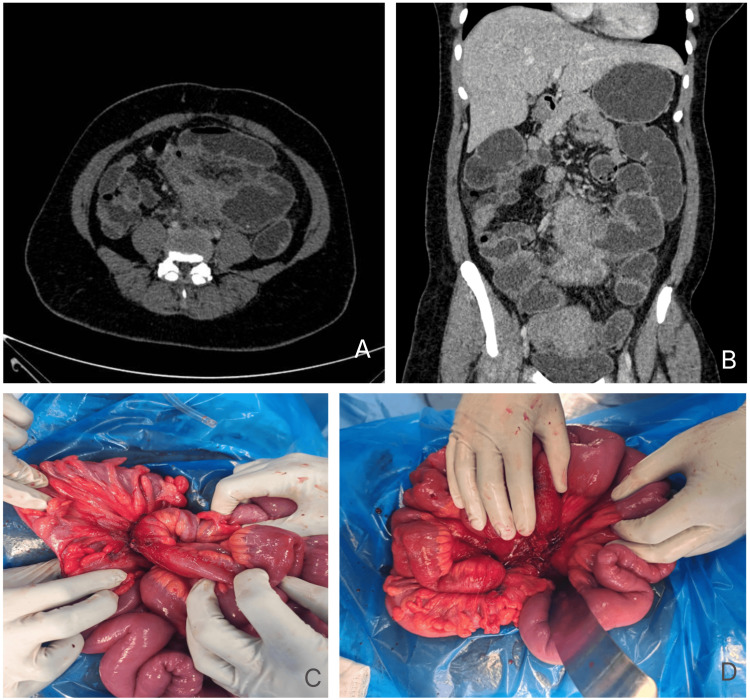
(A and B) CECT scan of the abdomen showed diffuse mural thickening involving a mid-ileal segment of approximately 6 cm, accompanied by luminal narrowing, surrounding inflammatory fat stranding, and an adjacent heterogeneously enhancing spiculated mass measuring 3.8 x 4.3 cm near the aortic bifurcation encasing the mesenteric root with prominent lymph nodes. (C and D) Terminal ileum was found to be densely adhered and clumped with the sigmoid colon and adjacent mesentery, forming a hard, ill-defined fibrotic mass at the umbilical level, just inferior to the aortic bifurcation CECT: Contrast-enhanced computed tomography
